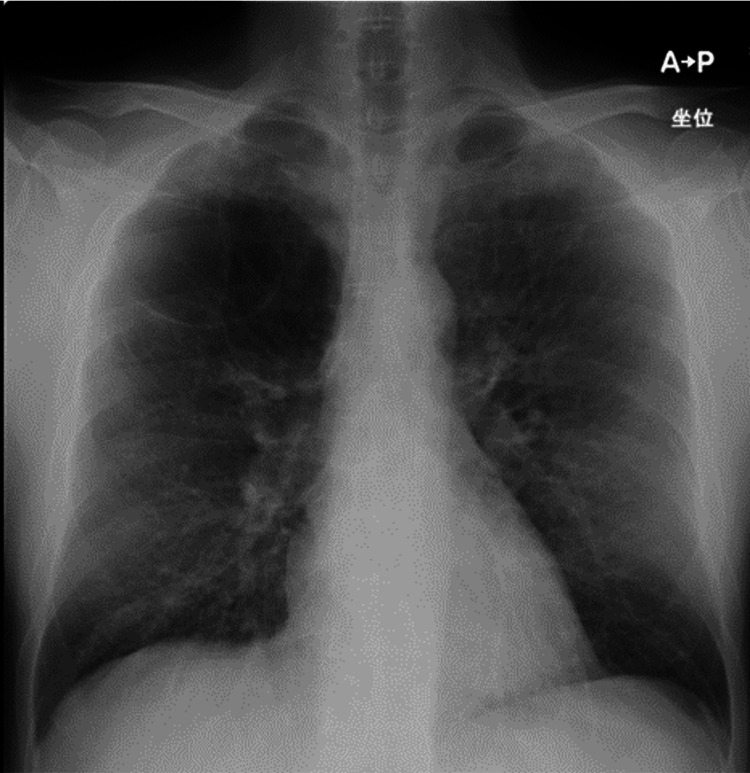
Chest X-ray on admission showed hyperinflation of both lungs
